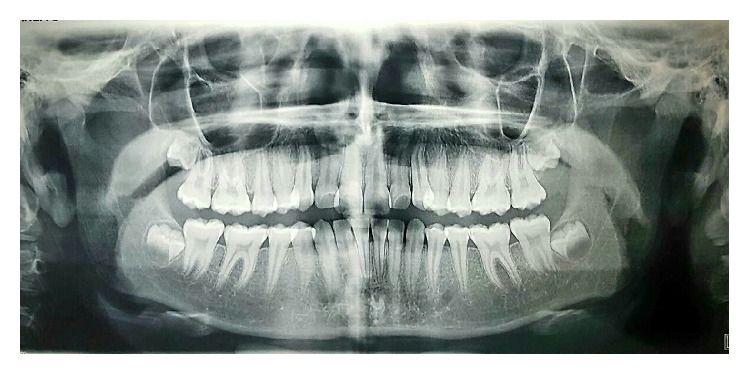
Pretreatment panoramic radiograph: the presence of all permanent teeth including the developing third molars.
